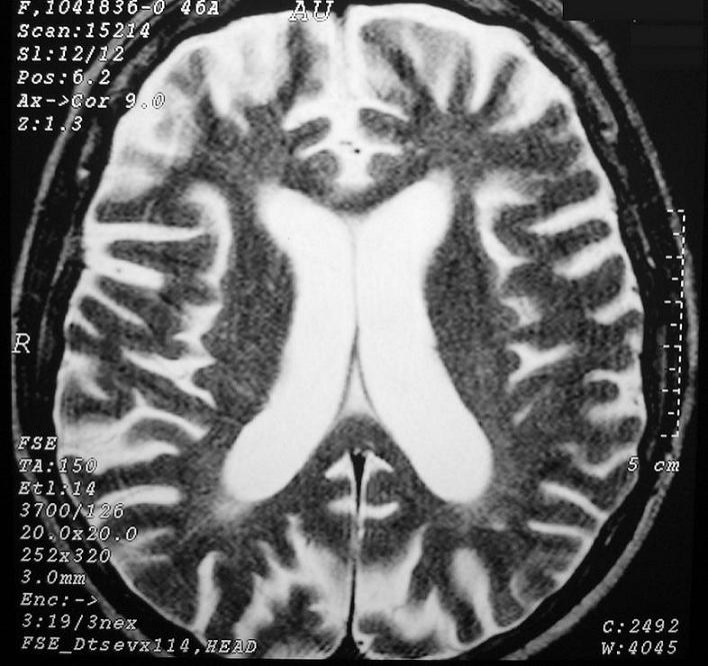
Brain axial T2W magnetic resonance imaging at the level of the lateral ventricles. Areas of high signal involving the periventricular white matter associated with enlargement of the lateral ventricles and widening of cerebral sulci.
